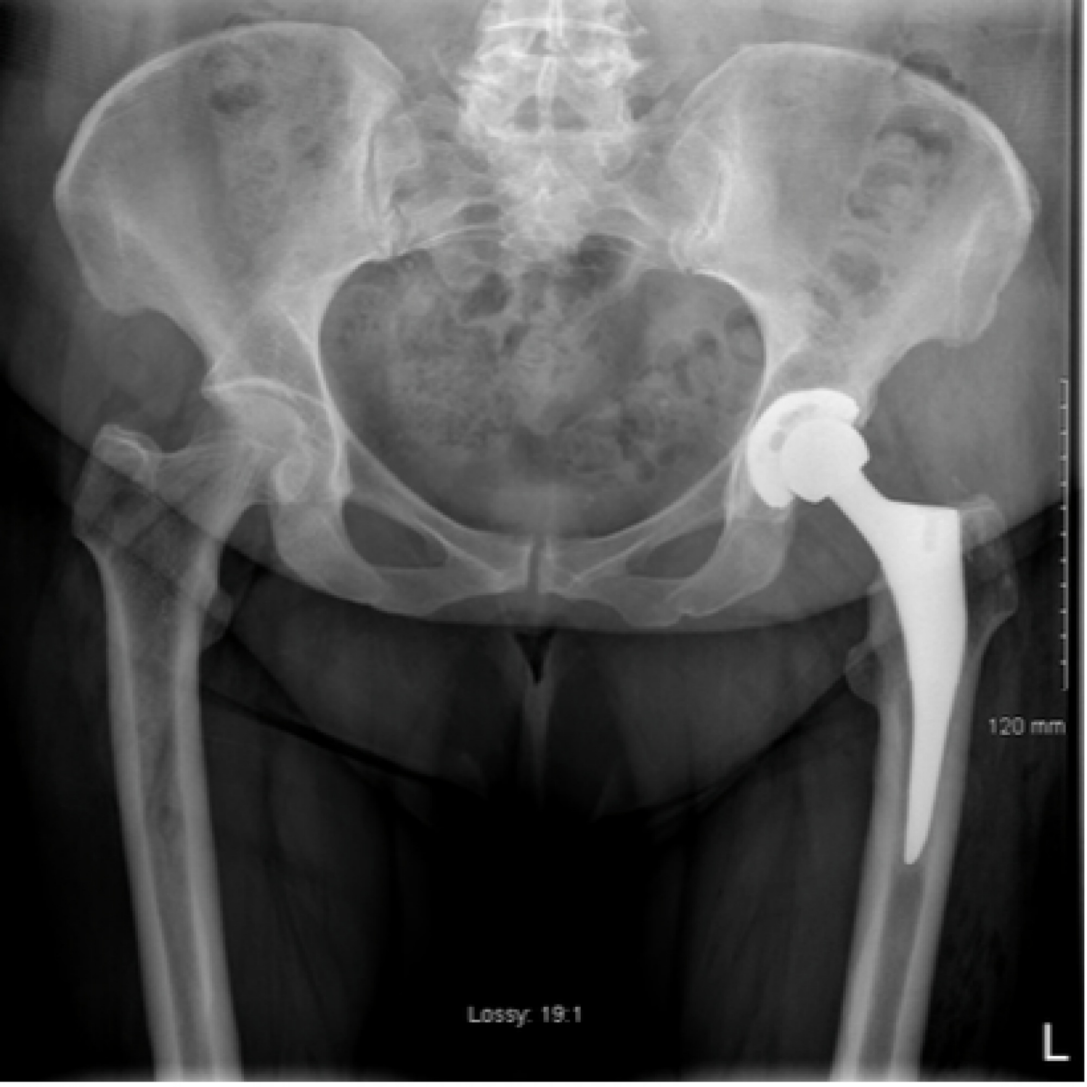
Post-operative AP radiograph of her left hip post head and liner exchange with concentric placement of the femoral head in the acetabular cup
